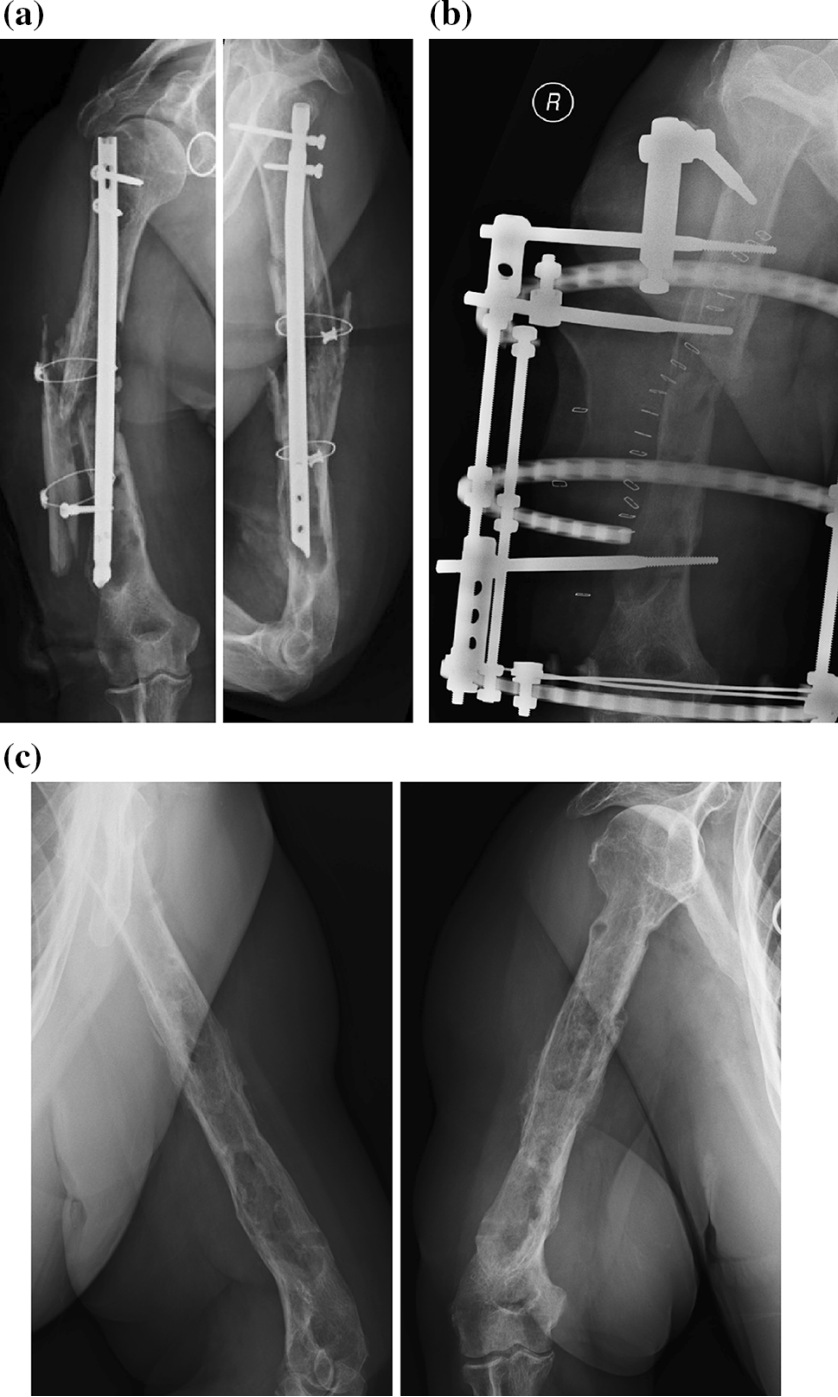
X-ray images of a case involving pre-operative instability (anatomical type IV infection). a This 72-year-old diabetic patient presented with a septic non-union of the humerus following multiple previous surgeries. b Reconstruction of the post-debridement defect involved acute shortening, bone graft, and circular external fixation. c Radiological images following removal of external fixator
